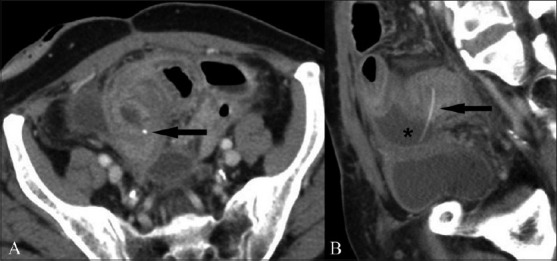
Axial (A) contrast-enhanced CT scan shows a small hyperdensity, which may be easily overlooked or confused with a vessel (black arrow in A), which however is very well appreciated in the sagittal reformatted image (arrow in B) penetrating through the bowel wall. Associated segmental bowel wall thickening is seen with presence of a small loculated collection (asterisk in B)
